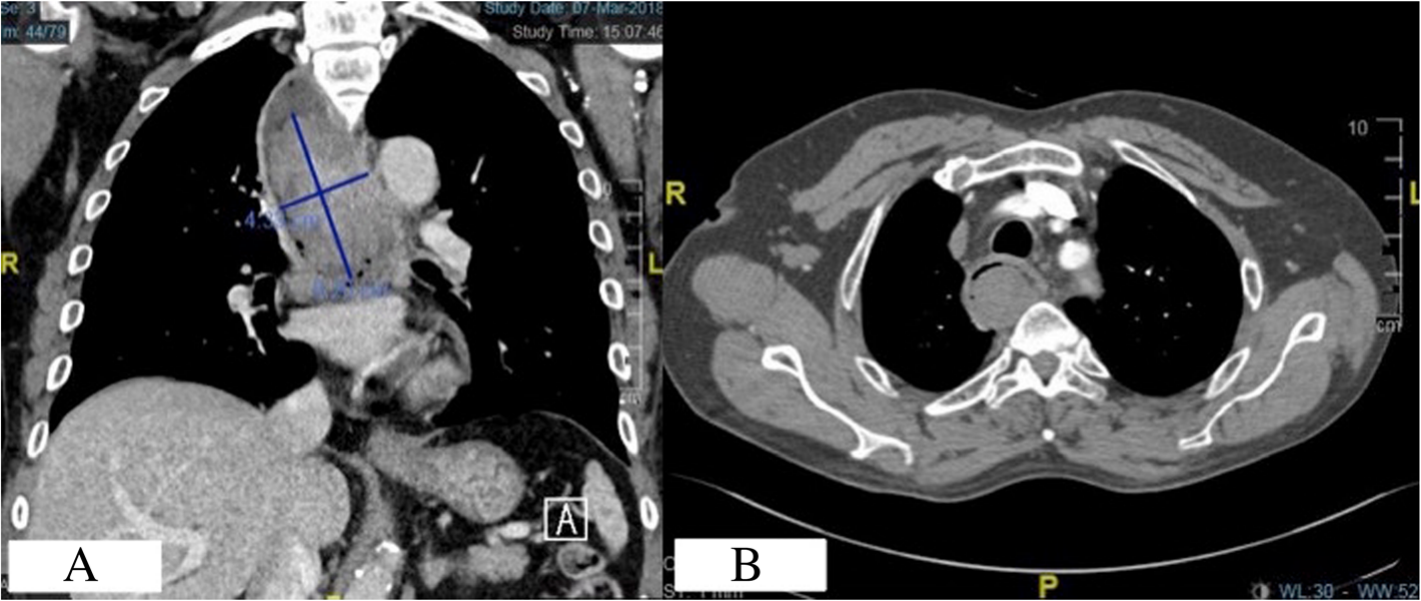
a, b Computed tomographic scan of the chest demonstrating soft tissue mass in the upper third of the oesophagus (coronal and transverse cuts)
